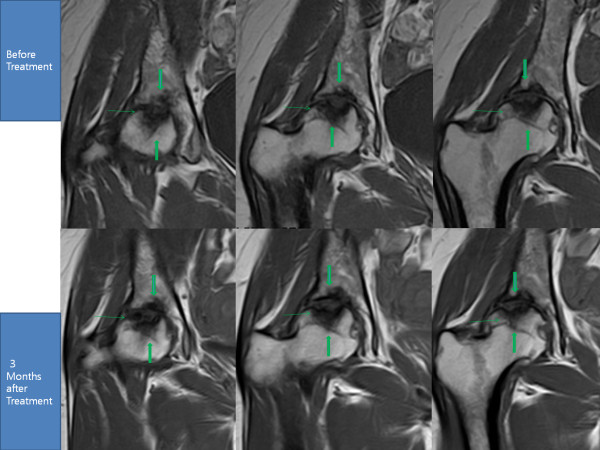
MRI of the right hip; T1 sequential coronal views. The cavity surrounded by the three green arrows has decreased in size in post-treatment MRIs due to probable bone regeneration.
